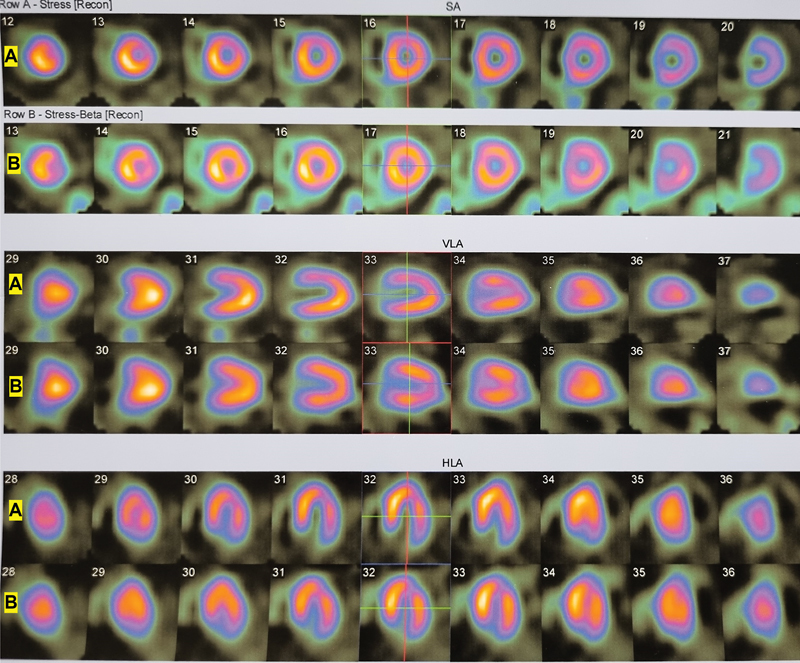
Stress phases of myocardial perfusion SPECT: (
